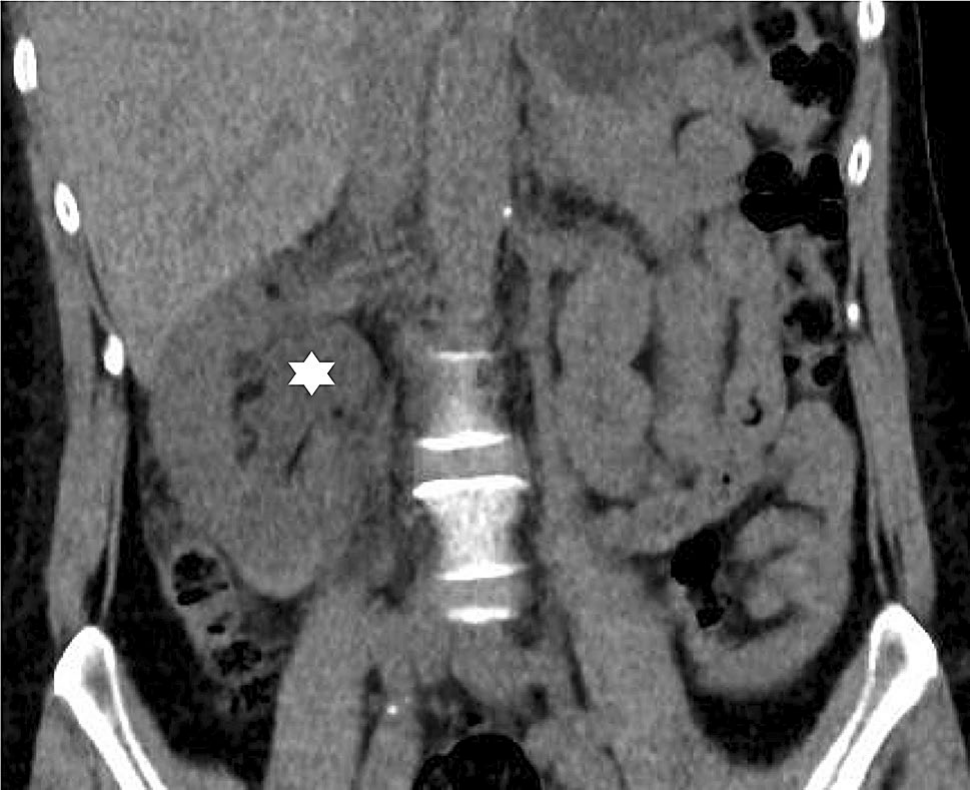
Non-contrast CT of the patient’s abdomen demonstrating right-sided hydronephrosis (asterisk)
